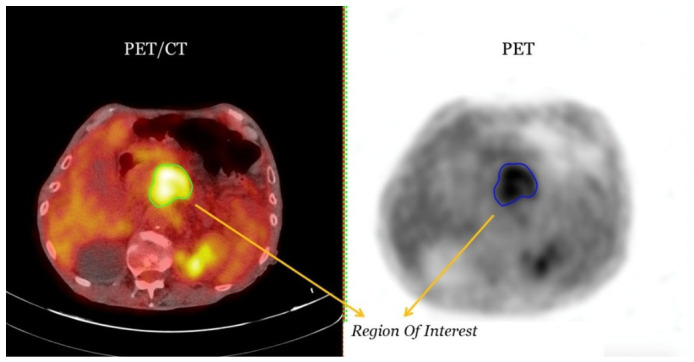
Transverse CT and PET scans of the same anatomical region. The segmented region of interest (ROI) can be seen in both images, enabling a direct visual comparison of anatomical and metabolic information.
